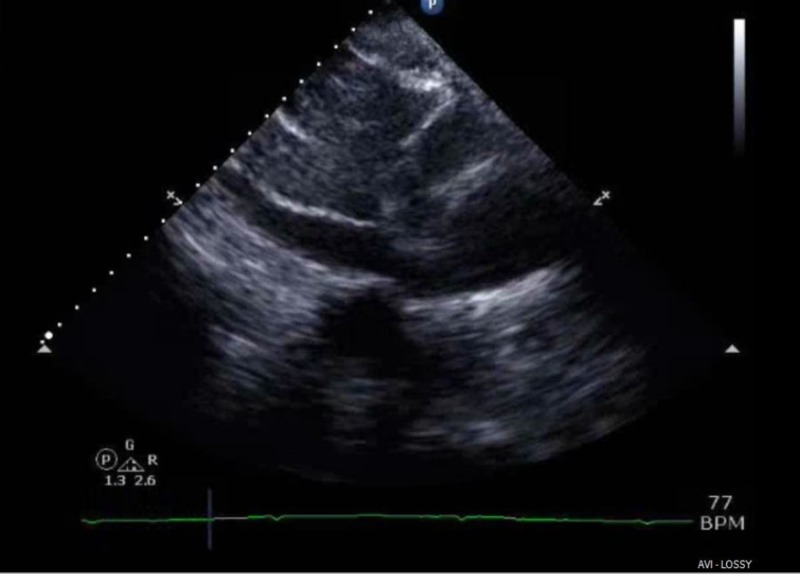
Echocardiogram; subcostal view. Revealing a dilated inferior vena cava (x) >2 cm with blunted respiratory variation indicating elevated central venous pressures.
